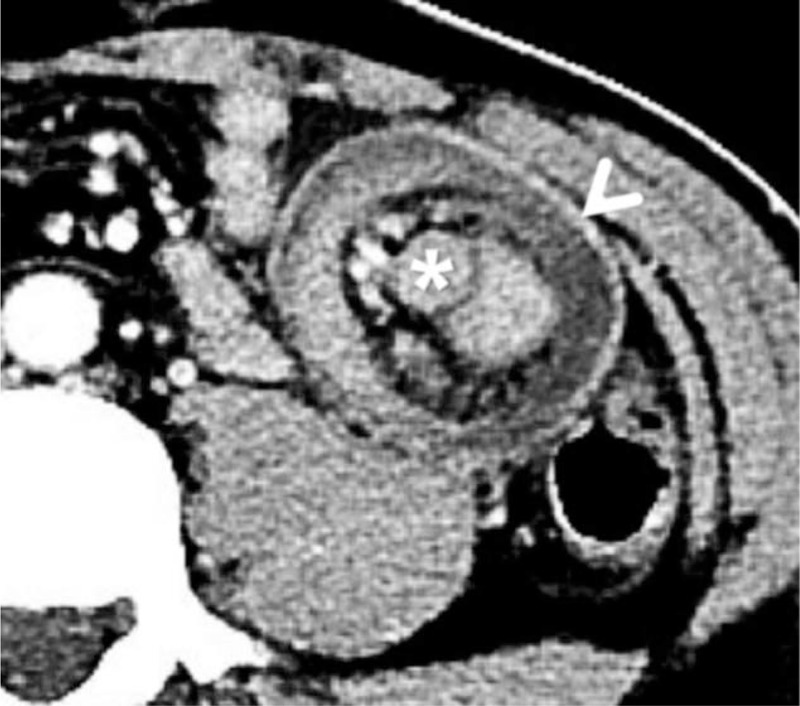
Axial section of computed tomography demonstrates a bowel-within-bowel configuration (arrowhead), and suspicious jejunal mass (asterisk).
